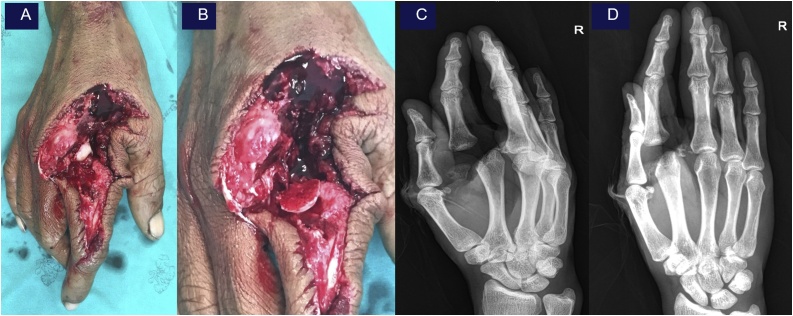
Pre – operative examination showing nearly amputation of right index (Fig. 1A). Radial of digital artery, vein and nerve of index completely tear with the articular surface of metacarpal head fracture (Fig. 1B). The radiographs show fracture dislocation of the head of the second metacarpal with articular involvement fracture 25% in oblique view (Fig. 1C) and AP view (Fig. 1D).
